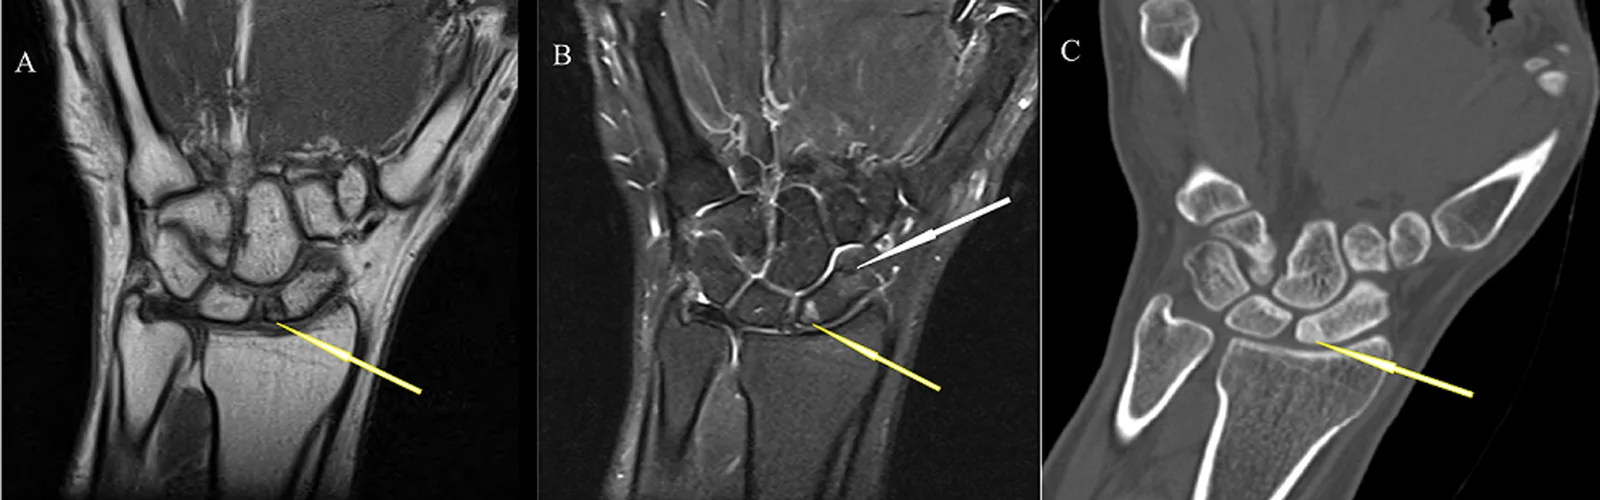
A) coronal T1-weighted MRI of the wrist shows a focal hypointense marrow signal abnormality in the proximal pole of the scaphoid (yellow arrow), suggestive of early ischemic change; B) coronal proton density fat-saturated MRI demonstrates a linear hypointense signal at the scaphoid waist (white arrow), consistent with a prior fracture site, with associated marrow edema; a focal hyperintense signal in the proximal pole (yellow arrow) raises suspicion for early avascular necrosis; C) coronal CT image shows apparent union of the scaphoid waist fracture with focal sclerosis of the proximal pole and no visible fracture line or collapse; these findings indicate structural healing, with sclerosis that may correlate with MRI-detected early vascular compromise
